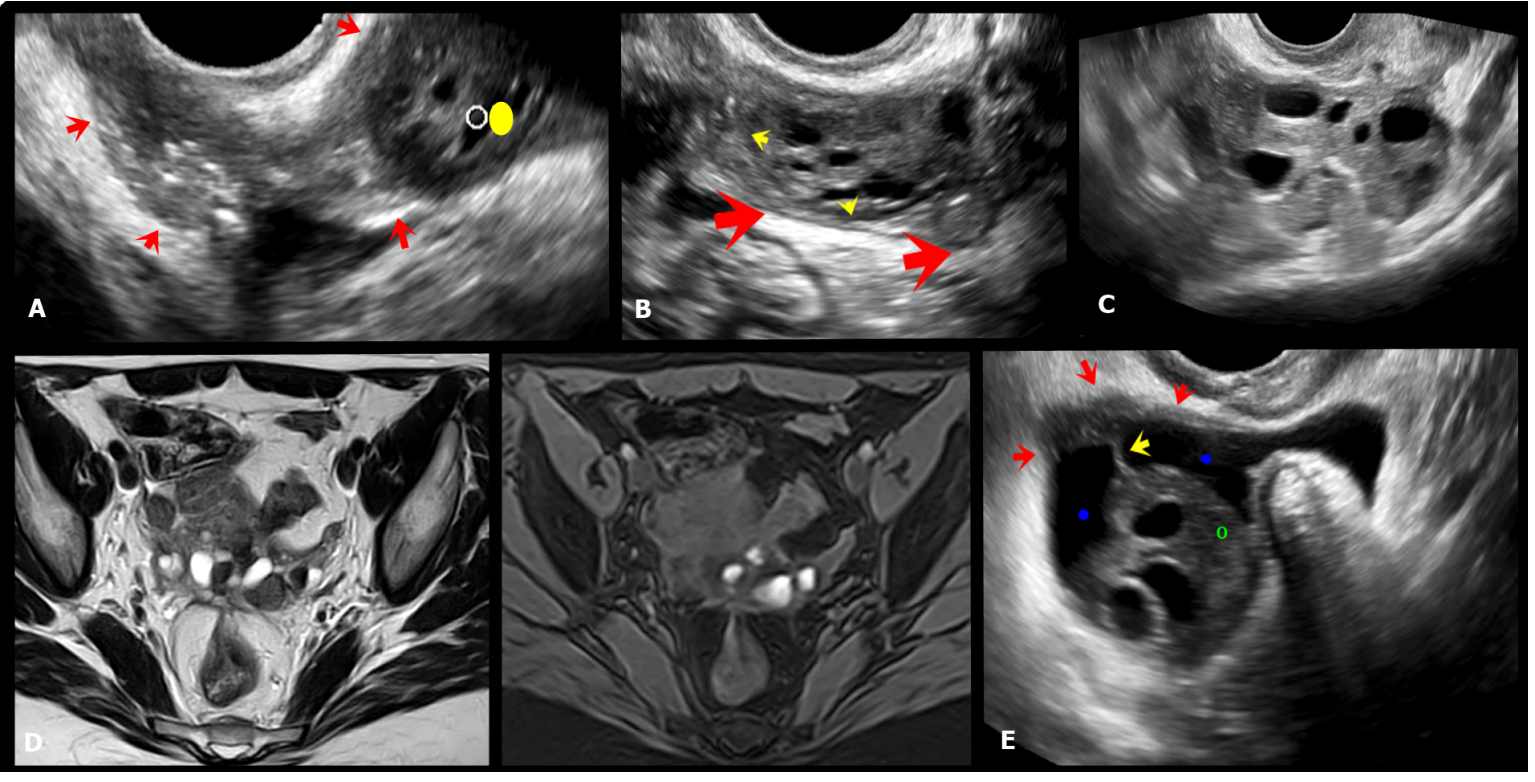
A. Axial oblique transvaginal ultrasound (TVUS) image shows a large hypoechoic endometriotic plaque (red arrow) with irregular and ill-defined margins, infiltrating the peritoneum of the left paracervical region and the ipsilateral ovarian fossa (yellow dot). B. Sagittal oblique TVUS image shows a hypoechoic endometriotic lesion infiltrating the left ovarian fossa (red arrows) and ovarian capsule (yellow arrows). C. Axial TVUS shows “kissing ovaries” as a result of posteriorisation and retraction of the ovaries out of the adnexa/ovarian fossae into the medial pelvis secondary to deep endometriosis (DE). D. Axial T2W and T1W MR images with fat suppression demonstrating kissing ovaries and multiple bilateral ovarian endometriomas and asd well as a DE plaque in the retrocervical space, involving the uterine torus, uterosacral ligaments and the serosa of the anterior wall of the rectum. E. Axial oblique TVUS image demonstrating indirect signs of the adherence process, with paraovarian inclusion cyst (blue dot) presenting thin septum (yellow arrow) and hypoechoic endometriotic lesion infiltrating the peritoneum of the ovarian fossa (red arrows).
